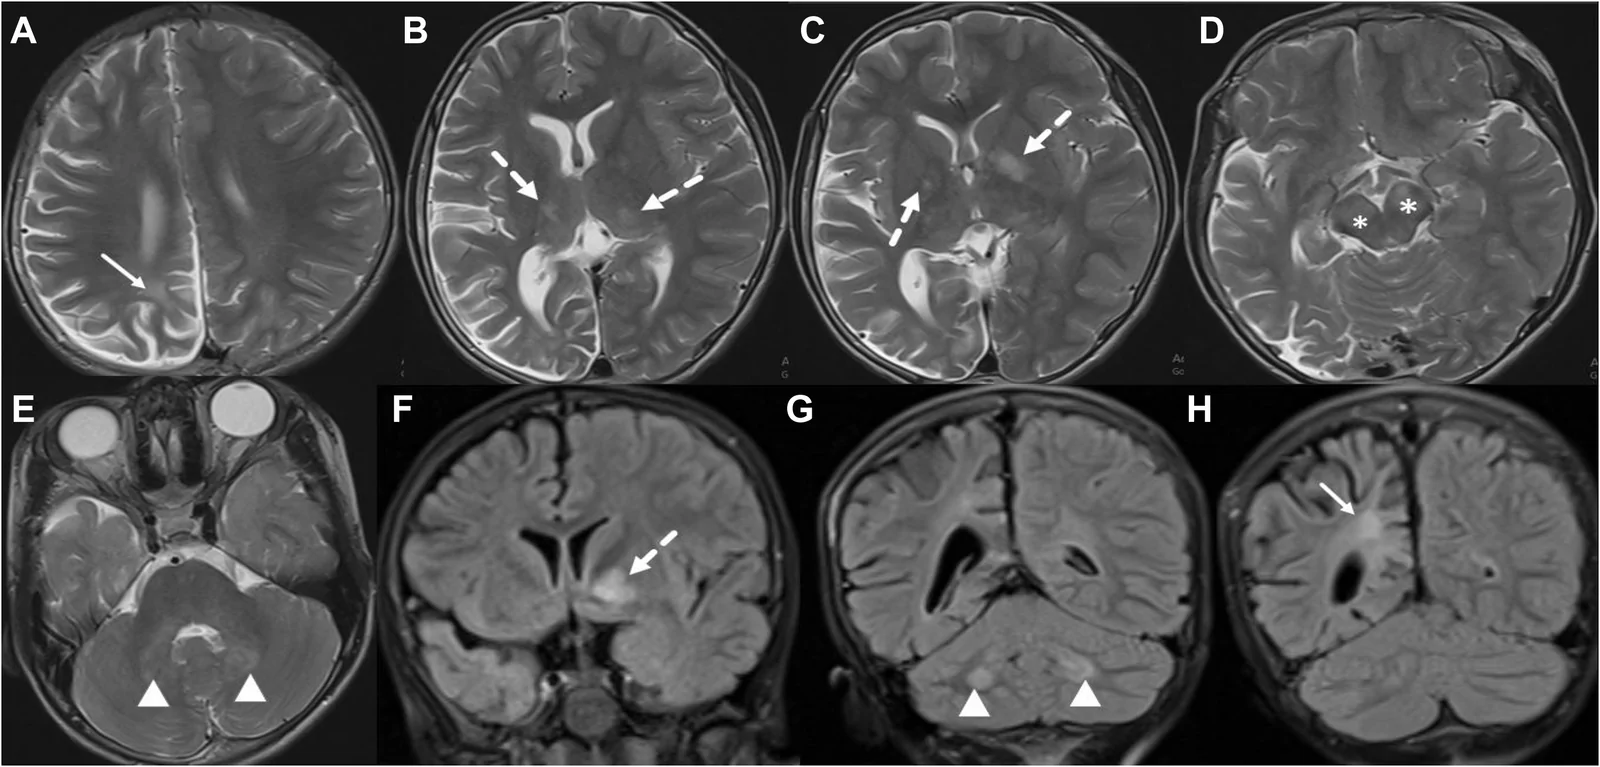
Non-contrast brain MRI of a 6-year-old boy with NF1 (A–H) shows axial T2/coronal FLAIR hyperintensities involving the deep white matter (*solid arrows*), deep gray nuclei, and thalami (*dashed arrows*), as well as the midbrain (*asterisk*) and dentate nuclei (*arrowheads*). *MRI,* magnetic resonance imaging; *NF1,* neurofibromatosis type 1.
